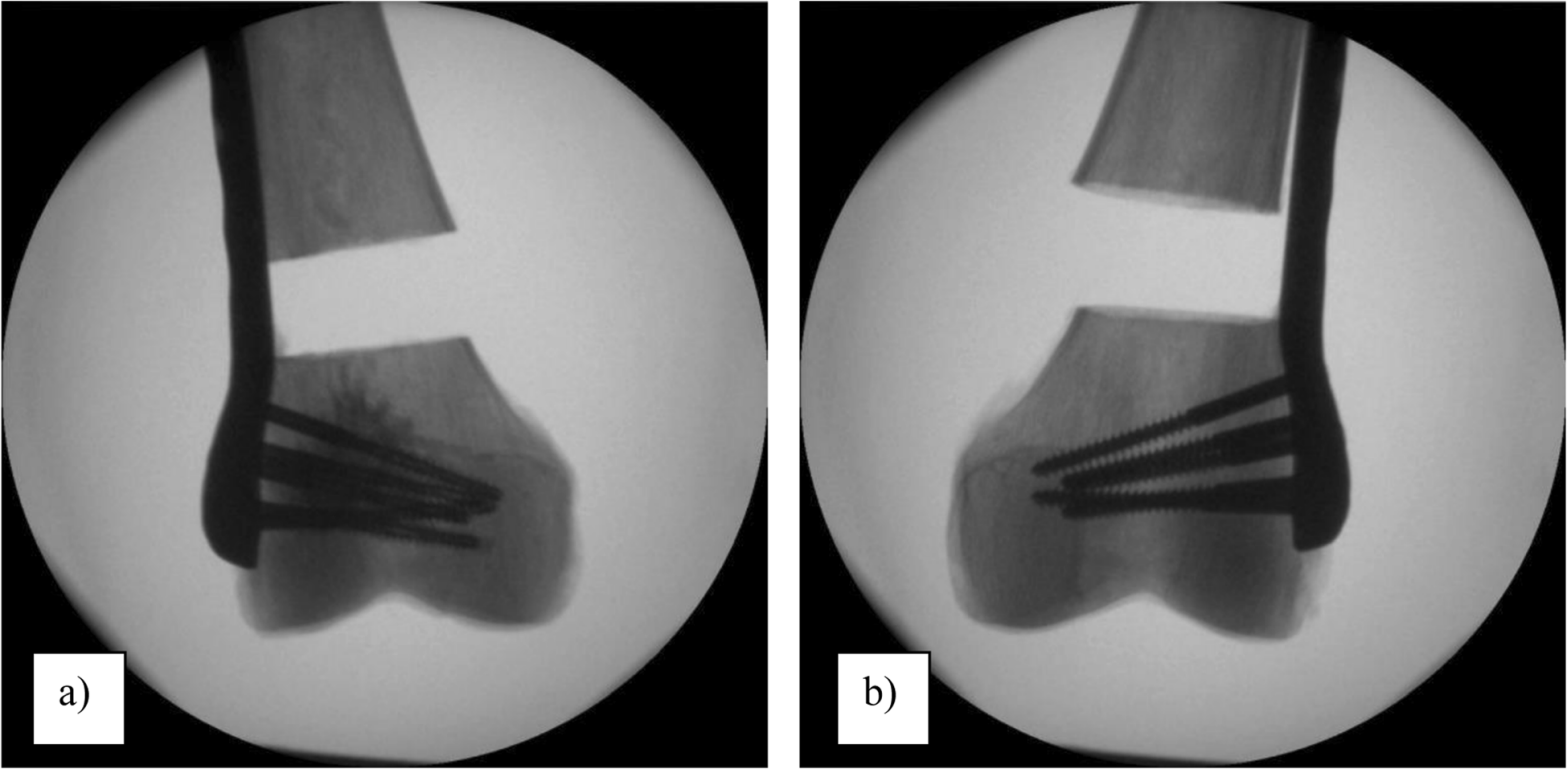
Typical postoperative X-ray pictures showing a pair of samples with cement augmented, fenestrated condylar screws in the right femur (a) and with non-augmented condylar screws in the left femur (b)
